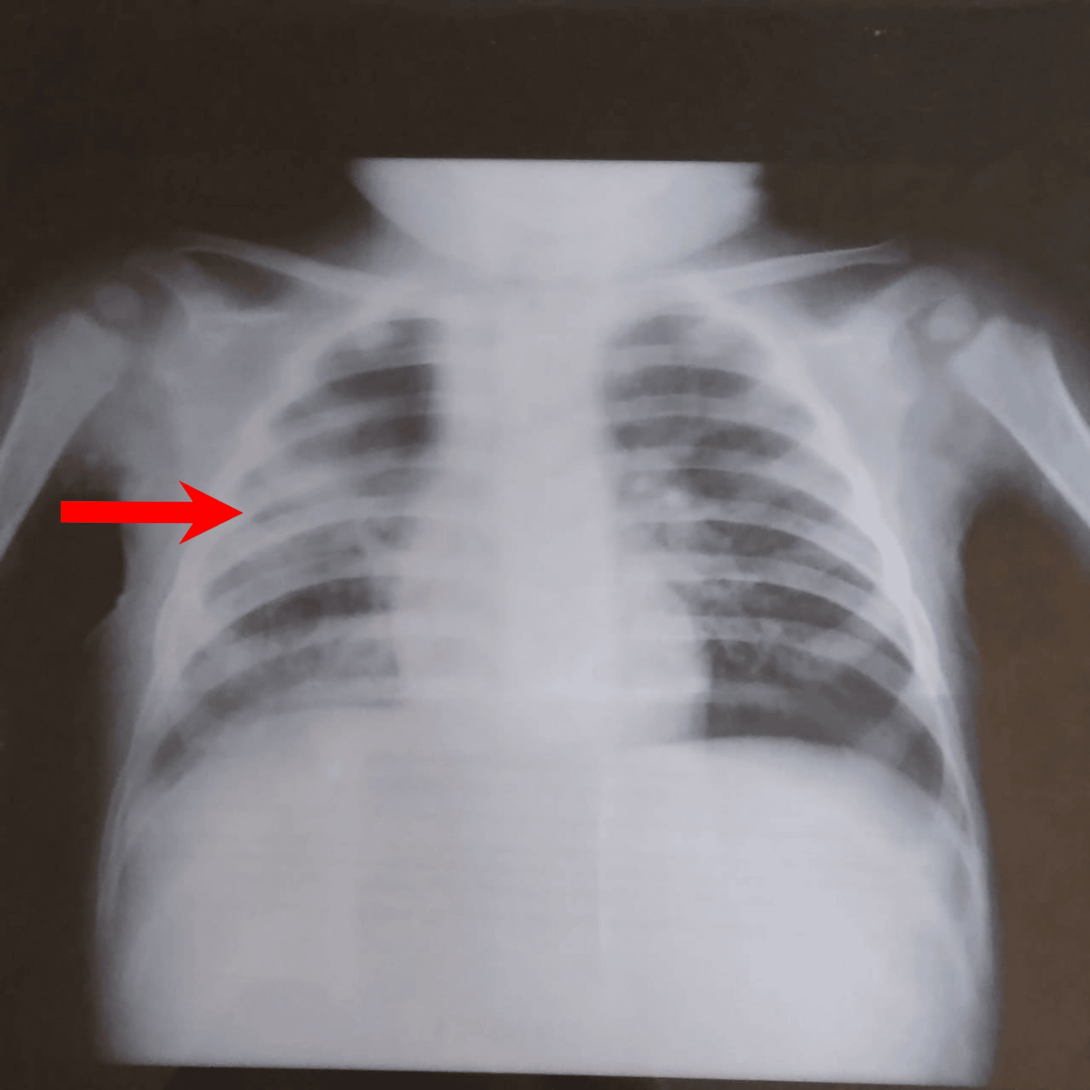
Frontal chest X-ray of the 2nd week post-operative.
